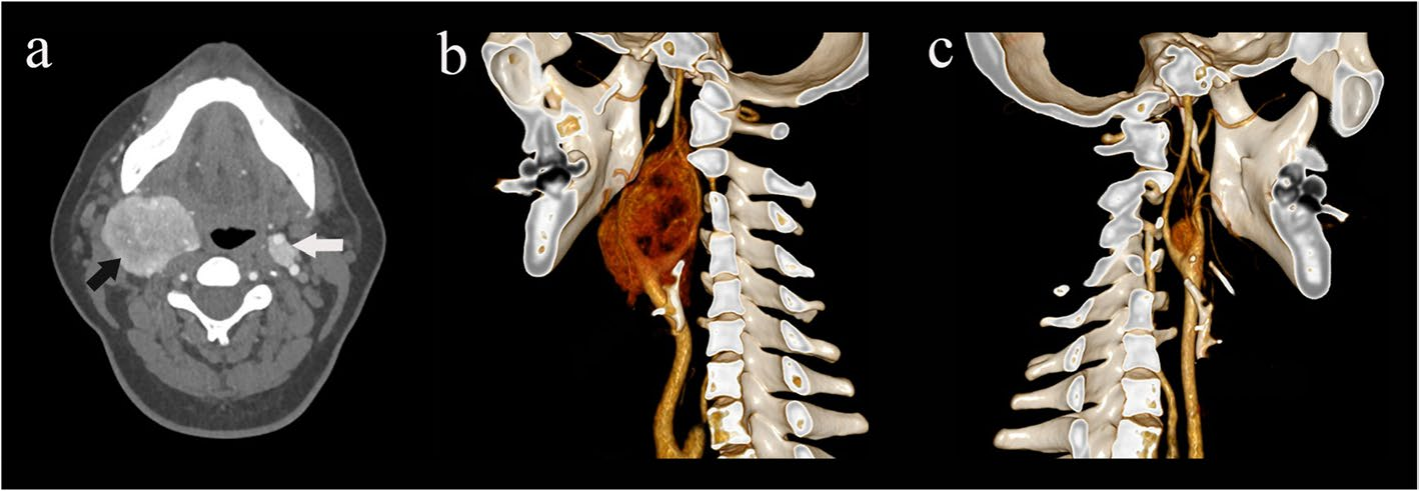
Images of a patient with bilateral CBTs. a preoperative transverse CT scan. Black and white arrows indicate CBTs on the right and left sides. b, c Preoperative CT angiography scan from both sides
